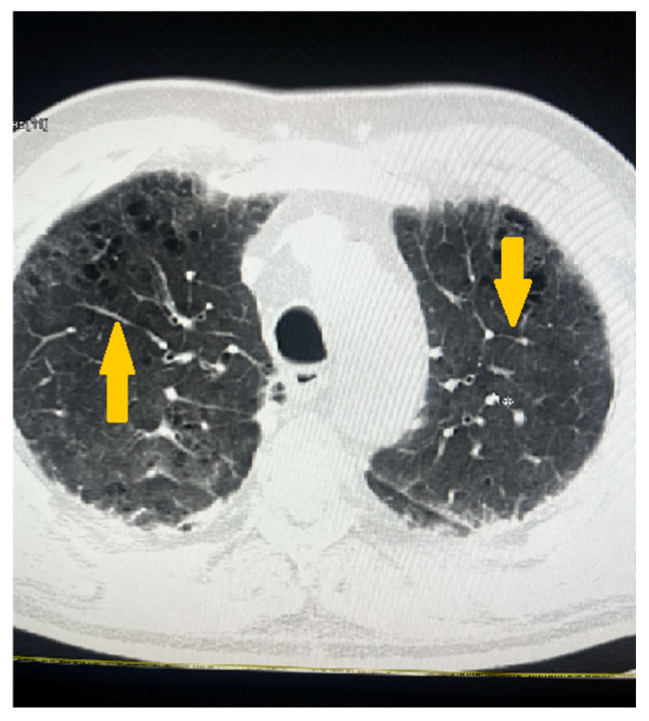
Non-contrast computed tomography (CT) showing the pulmonary parenchyma with prominent broncho-vascular markings (yellow arrows).
